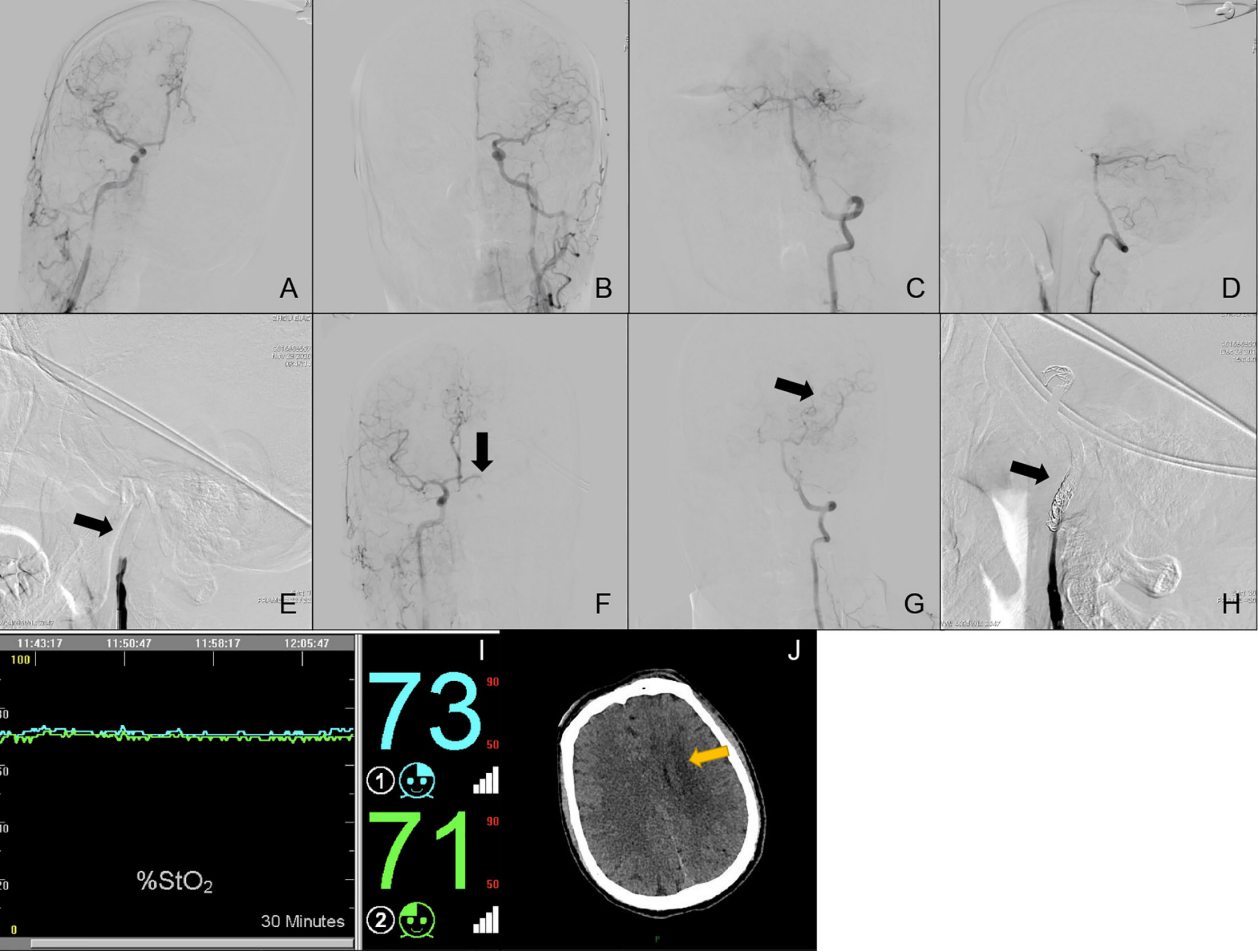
Cerebral angiography and cerebral rSO_2_ monitoring of Case No. 50. (A–H) Multi-side view of cerebral angiography. (I) Real-time rSO_2_ monitoring. The blue line represented rSO_2_ on the left cerebral hemisphere, the green line represented rSO_2_ on the right cerebral hemisphere. (J) CT scan of Case No. 50 after BTO and ICA occlusion, the yellow arrow indicates delayed cerebral infraction after therapeutic ICA occlusion.
